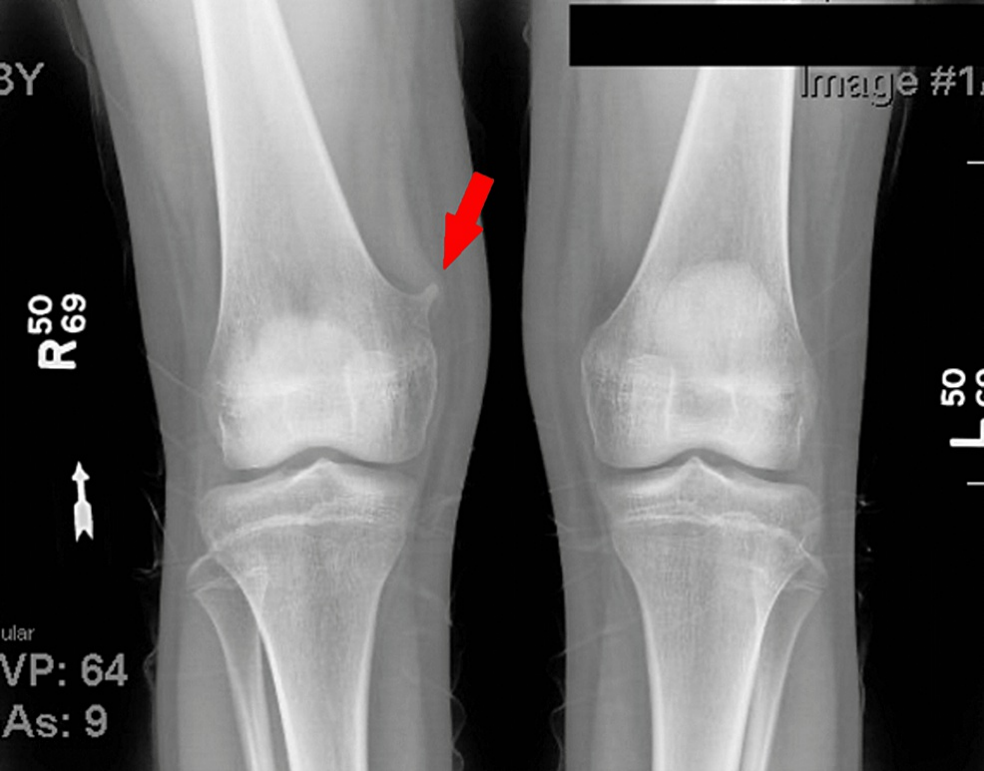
X-ray imaging revealing a bony exocytosis projecting superiorly from the right medial femoral condyle with features of an osteochondroma.
